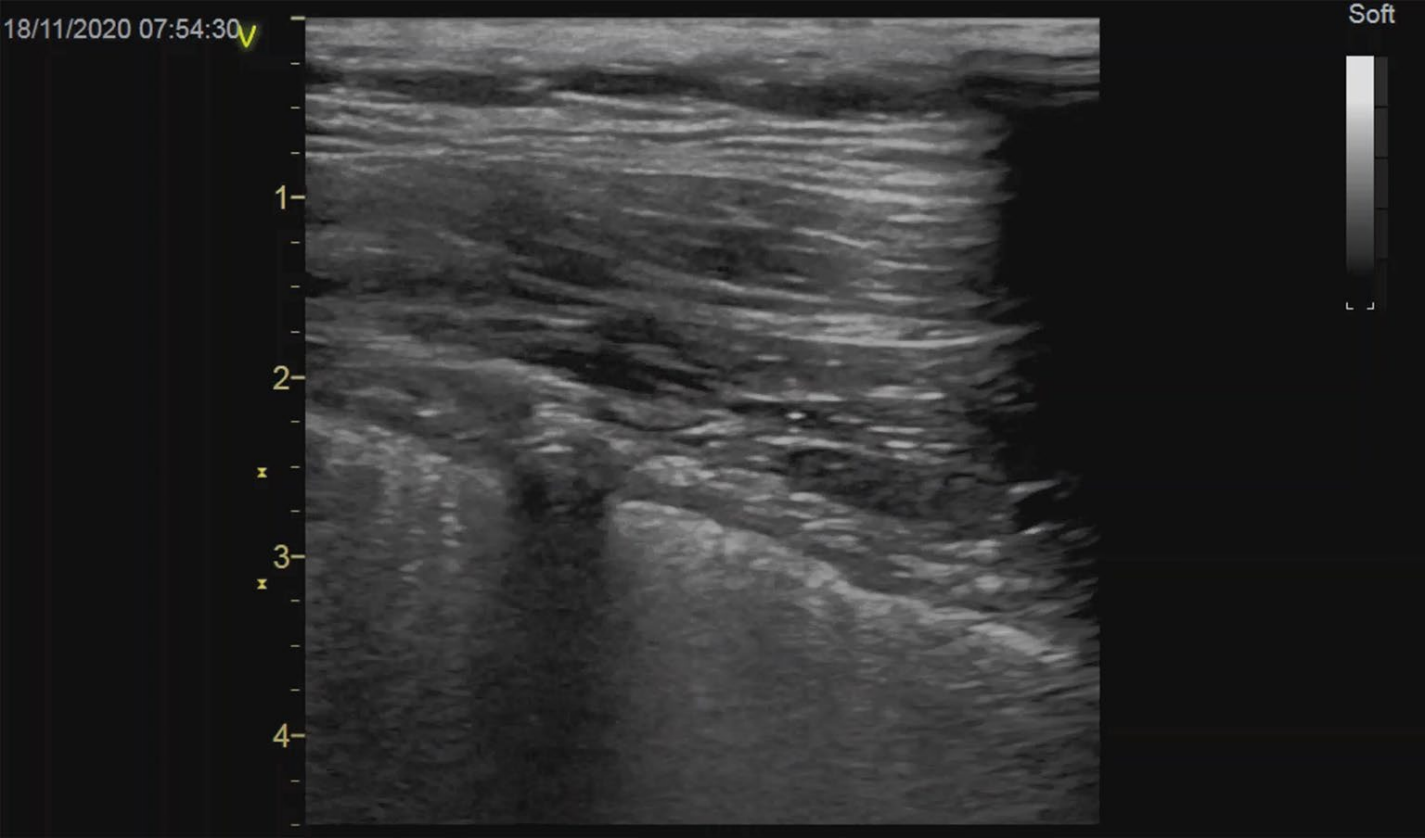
Chest ultrasound of the anterior chest wall in a Covid-19 ARDS patient after drainage of a pneumothorax due to barotrauma. The linear transducer depicts a chest drain in the transverse plane in between the enhancing visceral and parietal pleura
